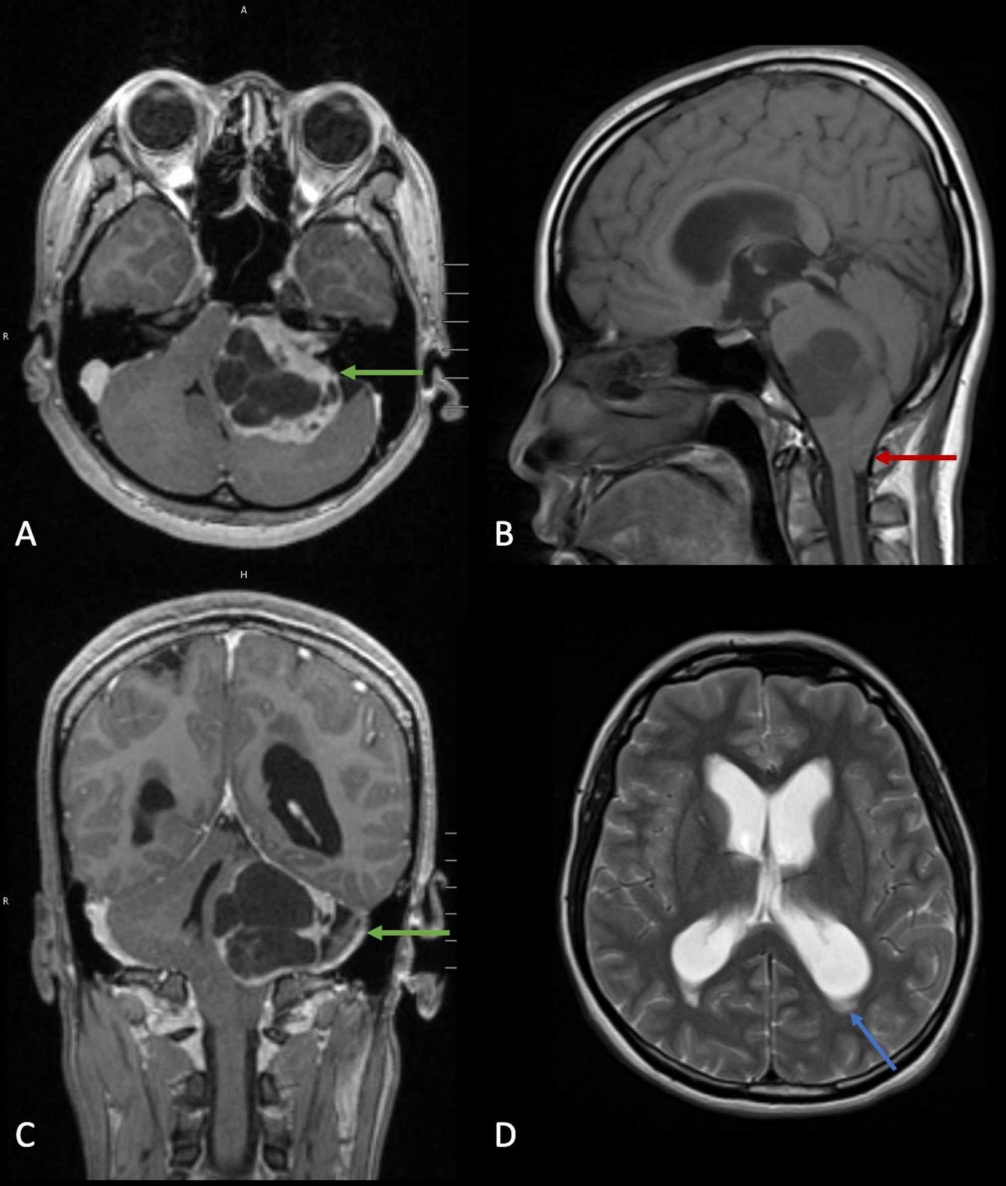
Preoperative MRI brain of large left-sided vestibular schwannoma in a patient who presented with false localizing right-sided isolated V2 sensory loss. (A) Axial T1-contrasted MRI showing a left-sided cystic heterogeneously enhancing Koos grade 4 vestibular schwannoma (green arrow). (B) Sagittal T1 MRI shows 1.7 cm tonsillar herniation (red arrow). (C) Coronal T1 contrast-enhanced MRI redemonstrates large cystic vestibular schwannoma with significant rightward brainstem displacement (green arrow). (D) Axial T2-weighted MRI shows enlarged lateral ventricles with transependymal flow (blue arrow).
